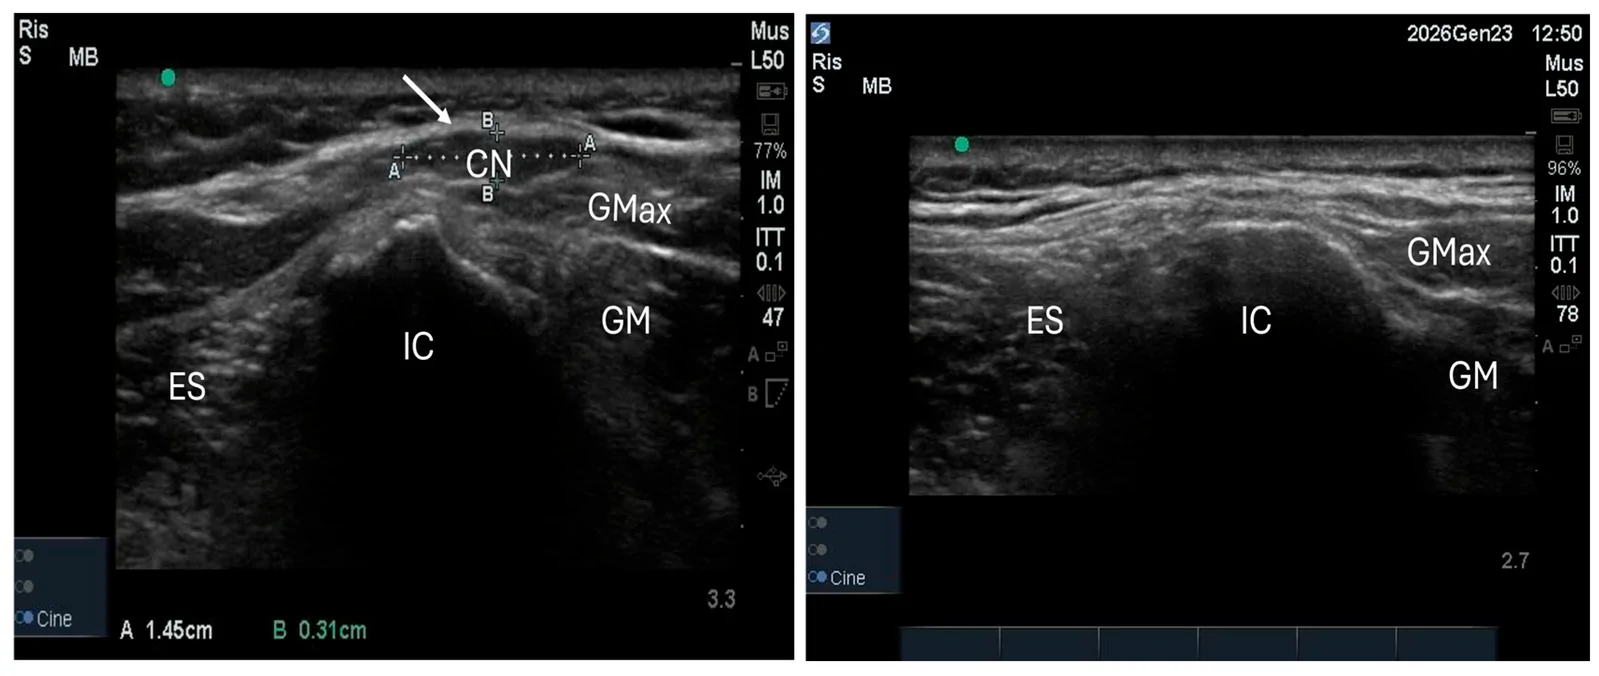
Ultrasound image of Copeman’s nodule (CN) in long-axis view. Identification of a CN (white arrow) in the lumbar region on the left, while the nodule is absent in the healthy control on the right. IC: iliac crest; GM: gluteus medius; Gmax: gluteus maximus; ES: erector spinae; CN: Copeman’s nodule. The letters A and B indicate the electronic calipers used for the dimensional assessment of the Copeman’s Nodule (CN).
